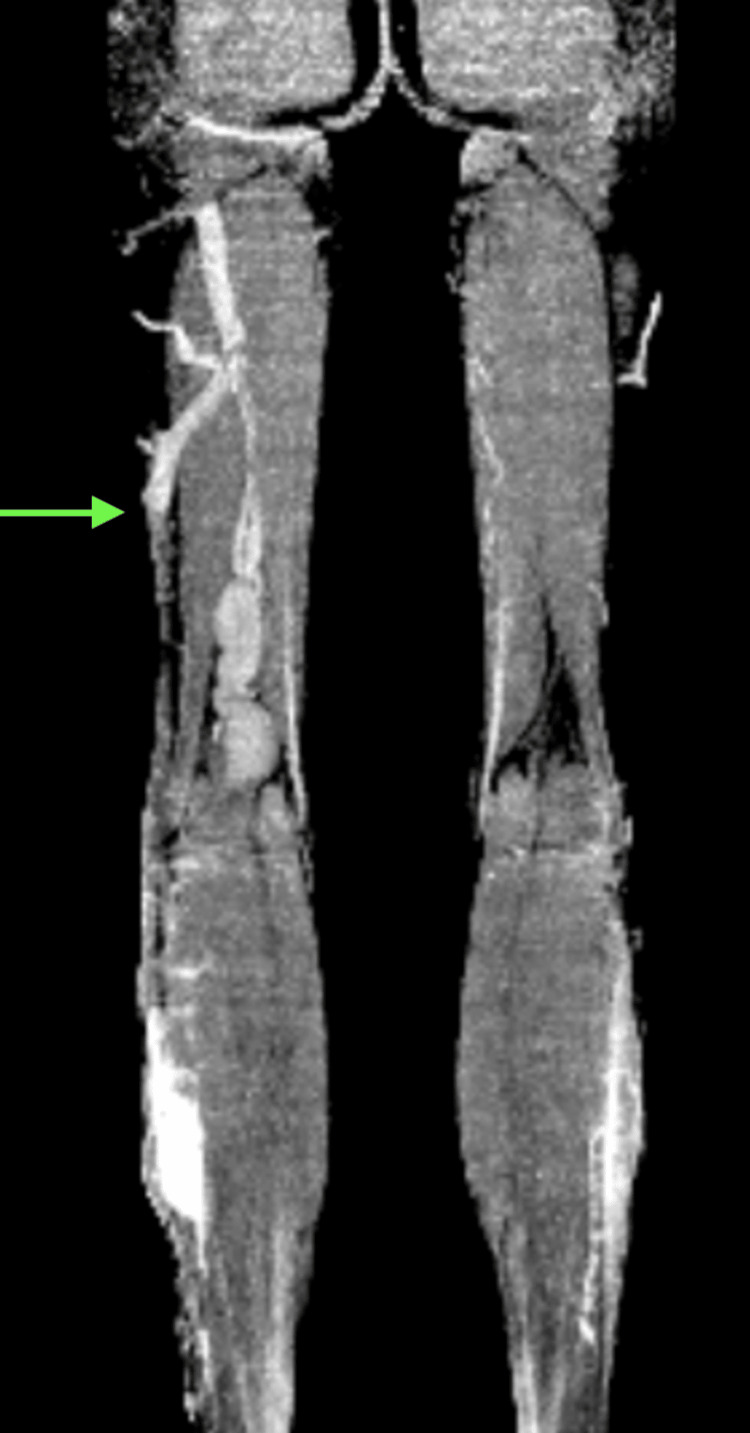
CT venogram of bilateral lower limbs showing the lateral marginal vein of Servelle (green arrow)
